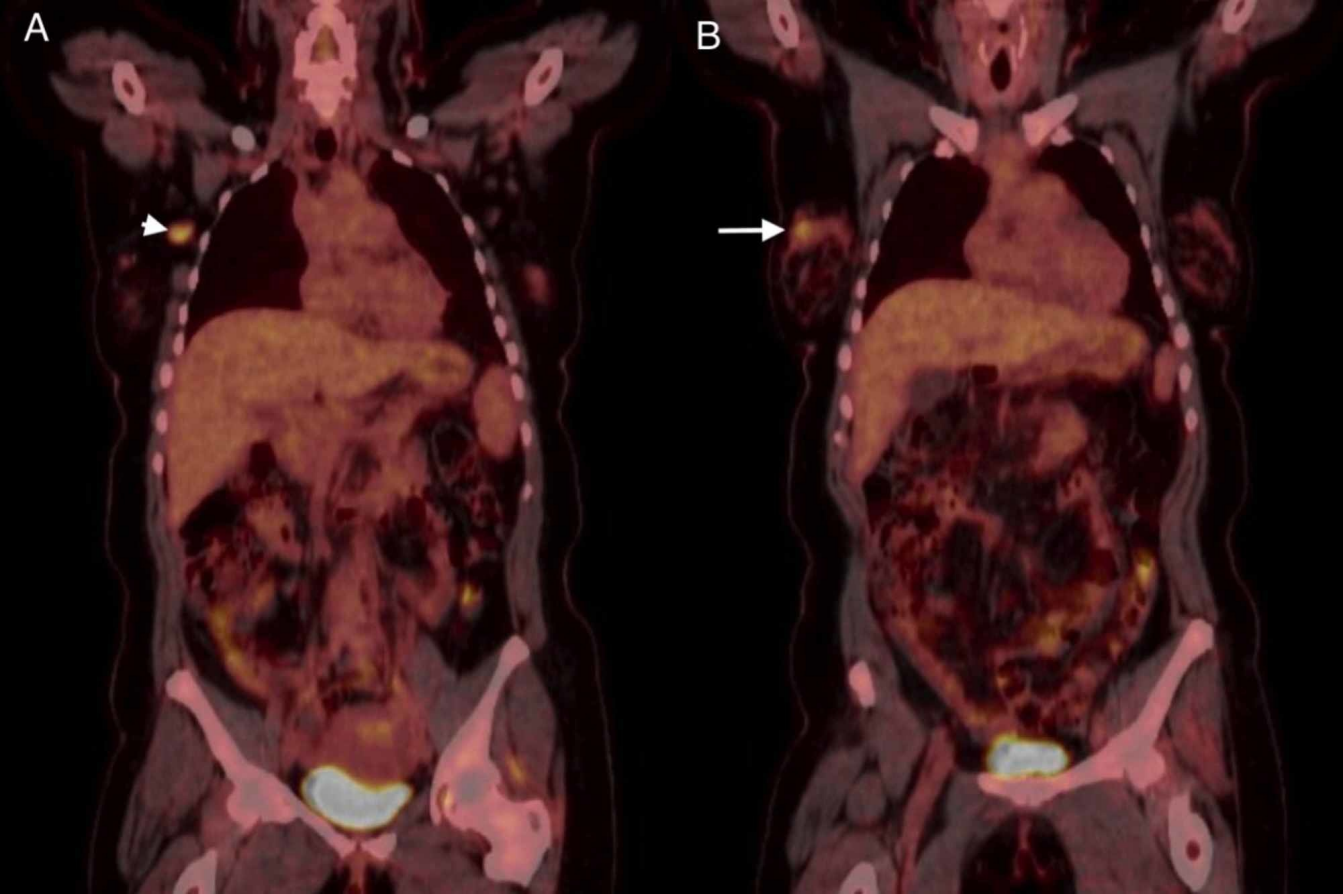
Fluorodeoxyglucose (FDG)-positron emission tomography-computed tomography (PET-CT) of the patient upon initial presentation. (A) The white arrowhead shows a focal region of increased FDG avidity in the lateral aspect of the right breast tissue. (B) The white arrow shows a single enlarged right axillary lymph node visualized posterior to the lateral chest wall measuring up to 2.5 cm in greatest axial dimension with avid FDG uptake.
